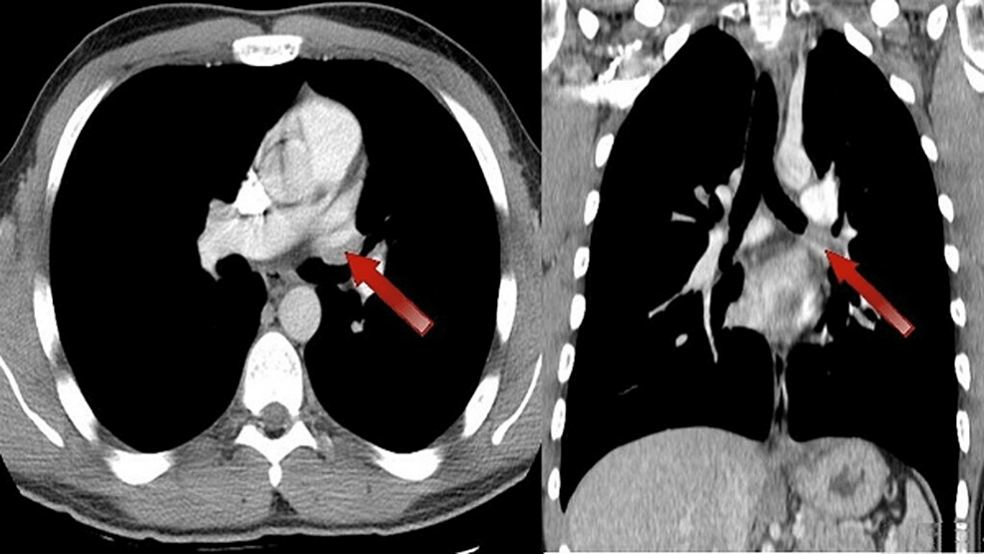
Axial and coronal CT images with contrast from June 26, 2020 (after four months of therapy) Further improvement in the mass, now measuring 2.0 cm x 0.5 cm (indicated by red arrows).
